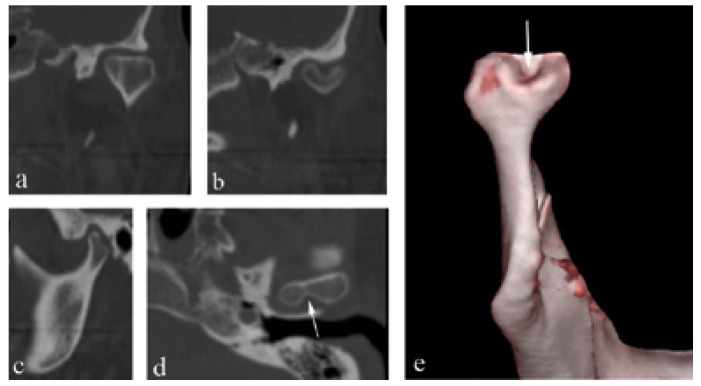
Coronal. a) CT scan of the patient (Patient-1) revealed no BMC. However, posterior slice, b) showed a clear BMC. Sagittal, c) CT scan showed irregular appearance of the posterior region of the condyle. Axial, d) CT scan showed a rift in the posterior of the condyle (arrow). 3D reconstruction image from posterior view, e) showed a partial BMC appearance and concavity between the duplicated condylar heads (arrow).
